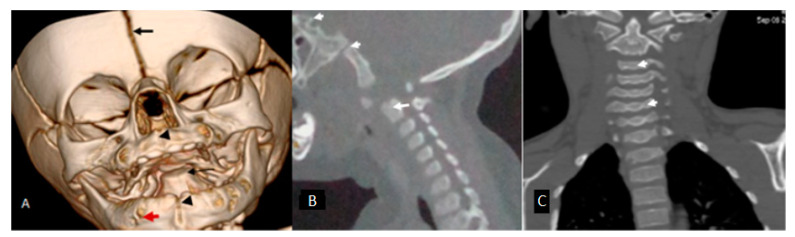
A 3D reconstruction CT scan of a 7-month-old girl with GS showed widely opened sutures, a short philtrum (pseudocleft lip), and midline clefting of the mandible (arrow heads). Agenesis of the odontoid process (arrow) and the multiple mandibular pits (red arrow) (A). A sagittal reformatted CT scan of a 20-month-old girl with GGS showed extensive anatomical disruption of the craniocervical junction, reflecting skull base synchondrosis—arrowhead. Dysplastic clivus, rudimentary atlas, and bifid dysplastic odontoid associated with dysplasia of the cervical vertebral bodies also note the thin and retarded closure of the posterior fontanelle-arrow (closure of the posterior fontanelle should take place after the age of 8 weeks of life) (B). A coronal reformatted CT scan of an 8-year-old girl with GS showed synchondrosis of C3-T1. The persistence of cervical spine and cervico-thoracic synchondrosis (arrow heads) can lead to progressive mal-alignment of the vertebral column, particularly in preadolescence (C).
